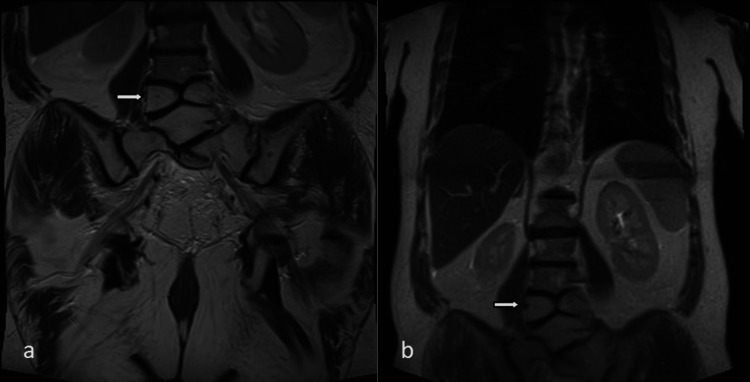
L4 hemivertebra in a 51-year-old male patient. Coronal T2-weighted image (a) and MR localizer sequence (b) show hemivertebra (arrows).
